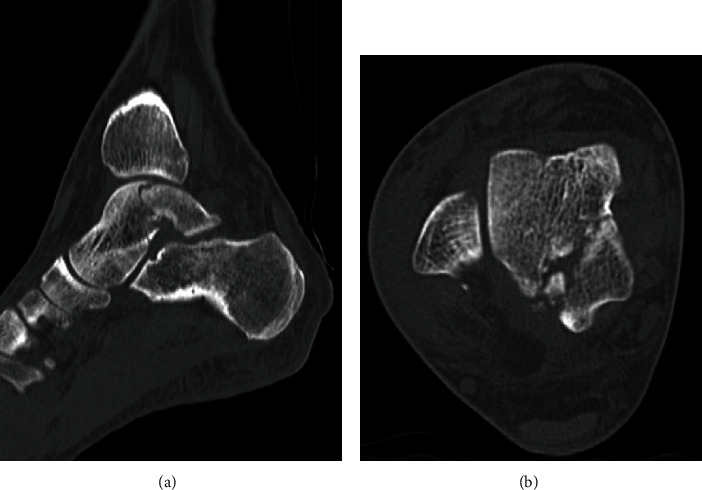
Preoperative noncontrast CT in (a) sagittal and (b) axial views demonstrated the fracture line from the lateral side of the posterior lateral process to the posteromedial side of the talus body. In addition, the fracture line extended to the posterior ankle and subtalar joints, and the bone fragment was slightly displaced.
